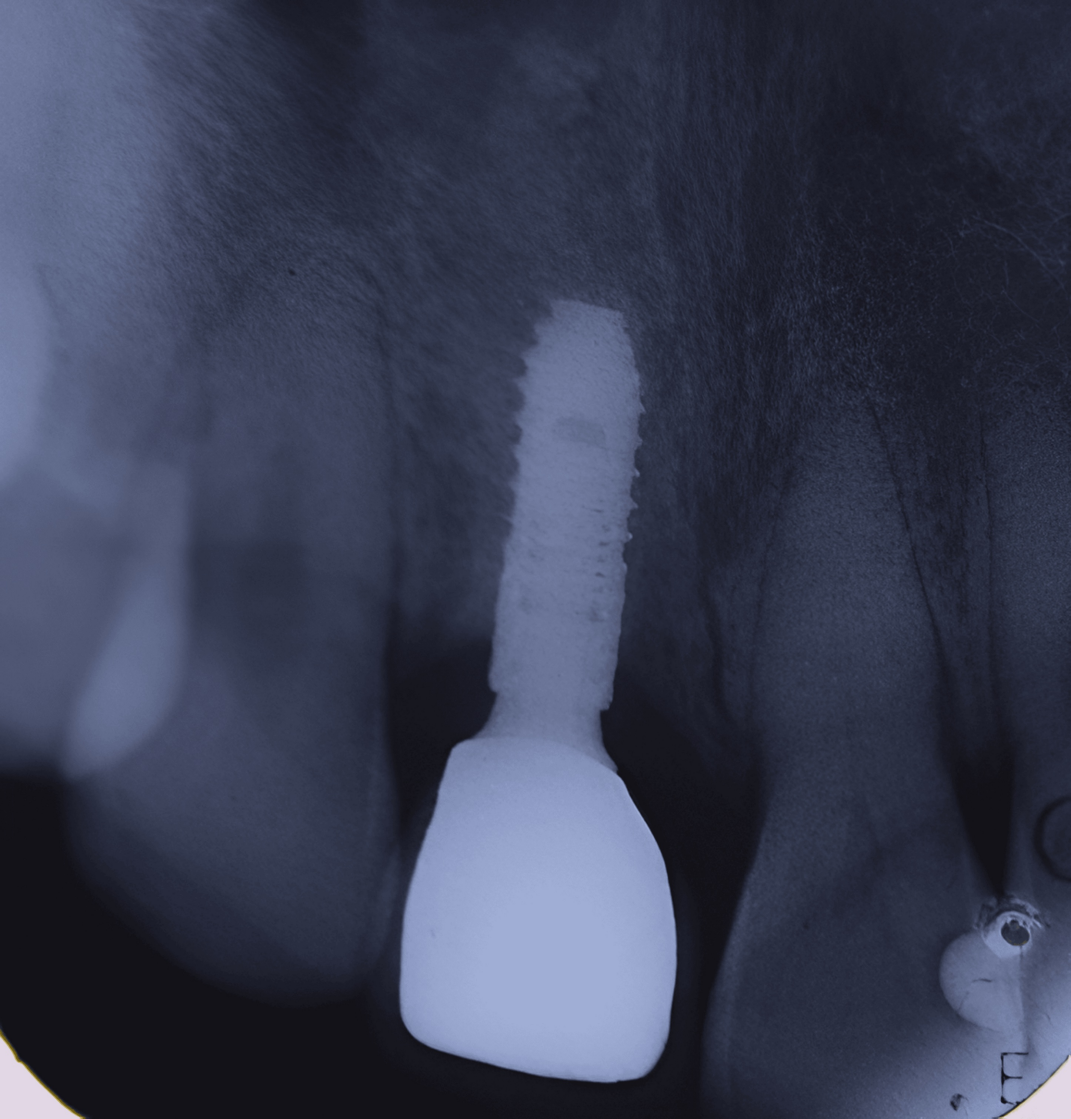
Case 3: IOPA taken during follow-up visit after six months showing successful functioning of the prosthesis without bone loss IOPA: Intra-oral periapical radiograph.
